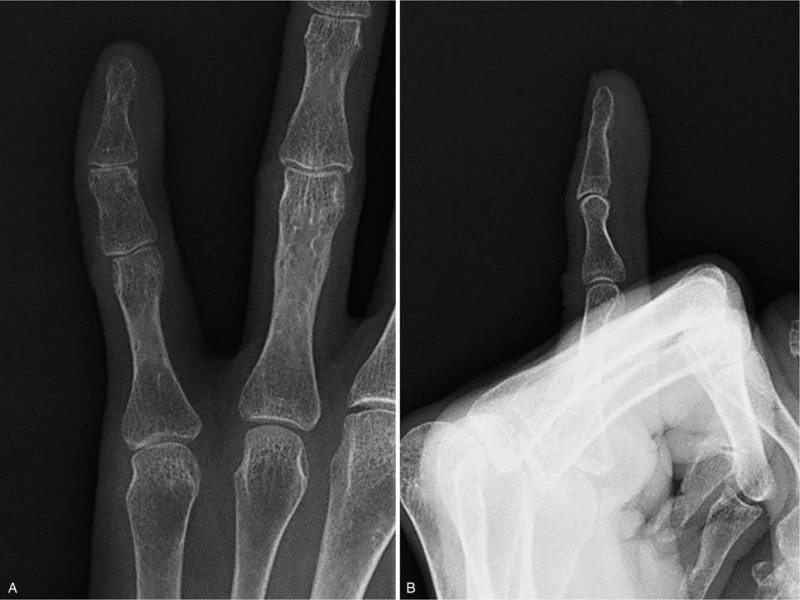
Plain (A) anteroposterior and (B) lateral radiographs taken 6 months after the initial presentation to our hospital, showing complete disappearance of the calcifying lesion around the proximal interphalangeal joint.
